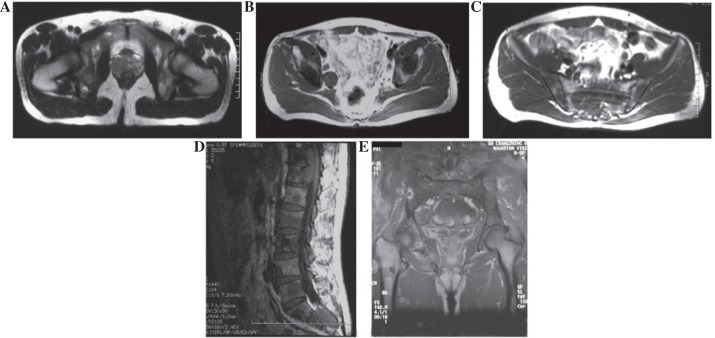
MRI of pelvis and lumbar spine (T1 weight). (A) Slightly increased prostate volume, with a less smooth fringe and non-uniform signal at the bottom of the peripheral ribbon. (B) Soft tissue nodules in the right groin. (C) Bilateral ilium showing a low signal, notably on the right. (D and E) T11–12 intraspinal tumor and bilateral thigh bone detecting low signals.
